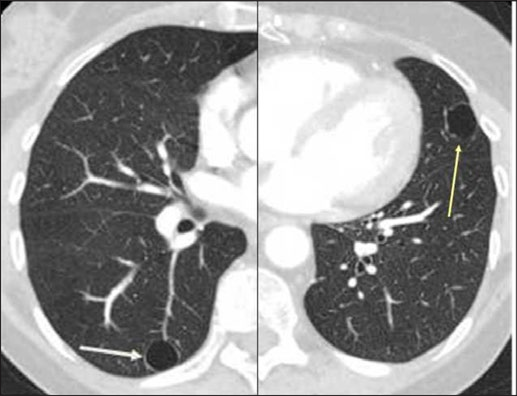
LIP.* A 71-year-old female with systemic lupus erythematosus for decades was admitted with increasing dyspnea and chronic cough, prompting a CT request. Thin-walled cysts are seen on the right and left sides (arrows). Diagnosis was LIP, which is associated with SLE.
